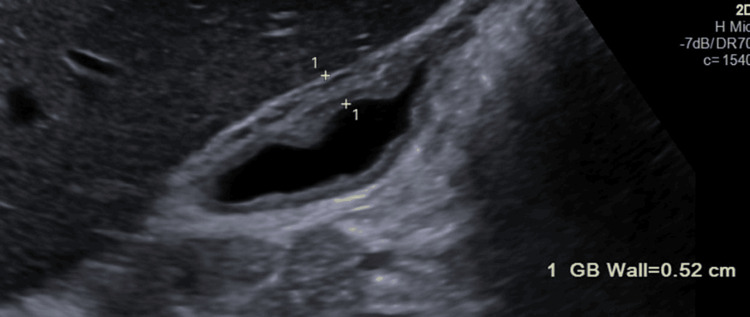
Right upper quadrant ultrasound demonstrating gallbladder wall thickening.
